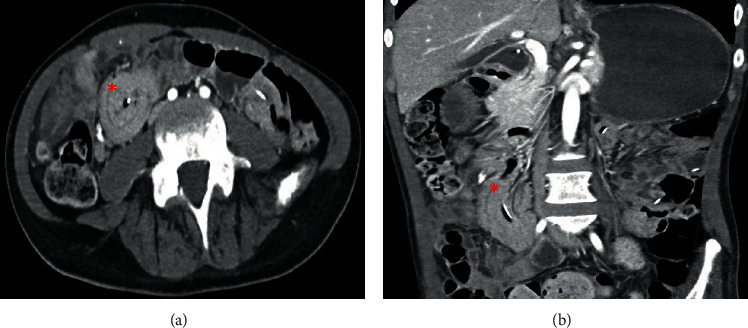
Axial and coronal computed tomography of the (a) abdomen and (b) and pelvis (with contrast) demonstrating enteroenteric intussusception at the junction of the second and third portions of the duodenum (red asterick) with the jejunostomy limb acting as the presumed lead point.
